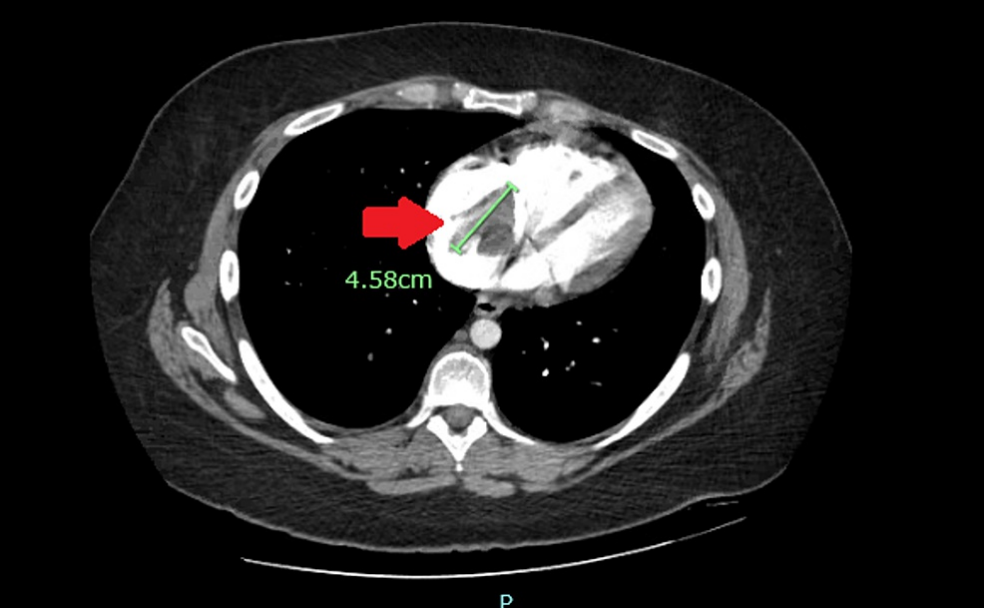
CT of the chest with contrast showing a 4.5 cm mass in the right atrium (red arrow). CT: computed tomography
